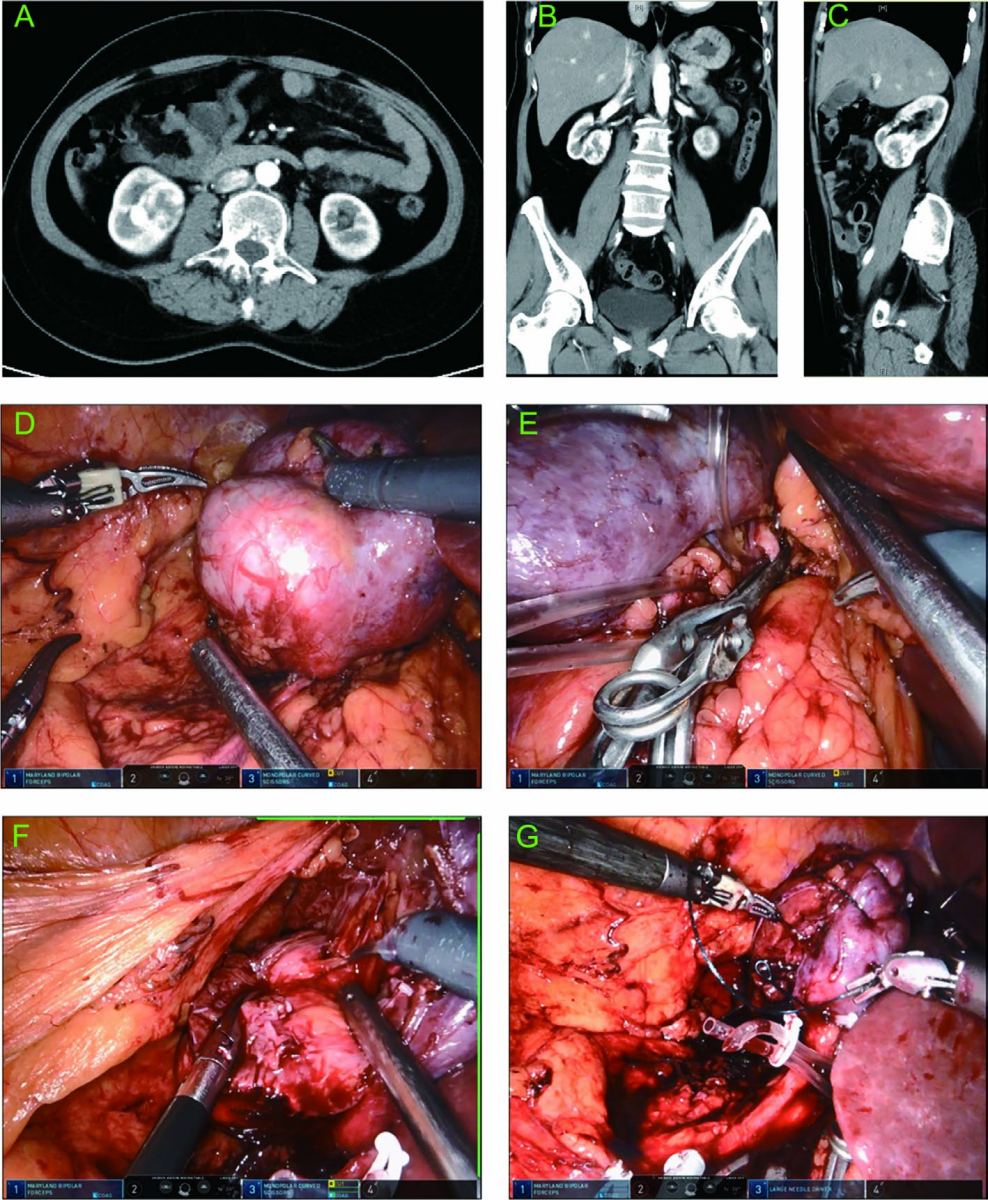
A typical case of the transperitoneal approach. (A–C) Contrast‐enhanced CT demonstrated an enhanced‐density mass measuring approximately 4.6 cm; (A), (B), and (C) showed the horizontal, coronal, and sagittal images, respectively. (D) The tumor was well exposed in the peritoneal cavity with a clear field of view. (E) The renal artery was clamped. (F) The tumor was removed using a robotic arm, and part of the collecting system was resected. (G) The wound after tumor resection was sutured.
